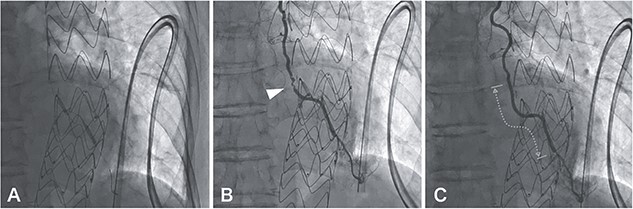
A 0.014-inch guidewire (SION Blue, Asahi Intecc, Aichi, Japan) was inserted into the LITA graft under intravascular ultrasound (Altaview; Terumo, Tokyo, Japan) guidewire; the diameter of the lesion was about 2 mm, therefore balloon dilation (Ryurei 2.0 × 20 mm, Terumo, Tokyo, Japan) was performed first from distal to proximal (A); angiography showing hematoma formation in the lesion, which was not resolved by the administration of nitroglycerin (B, arrowhead); three stents (Synergy 2.25 × 12 mm, 2.25 × 28 mm, 2.25 × 20 mm, Boston Scientific, USA) were overlappingly placed to cover the lesion (C, dotted line), and there was a satisfactory angiographic result.
